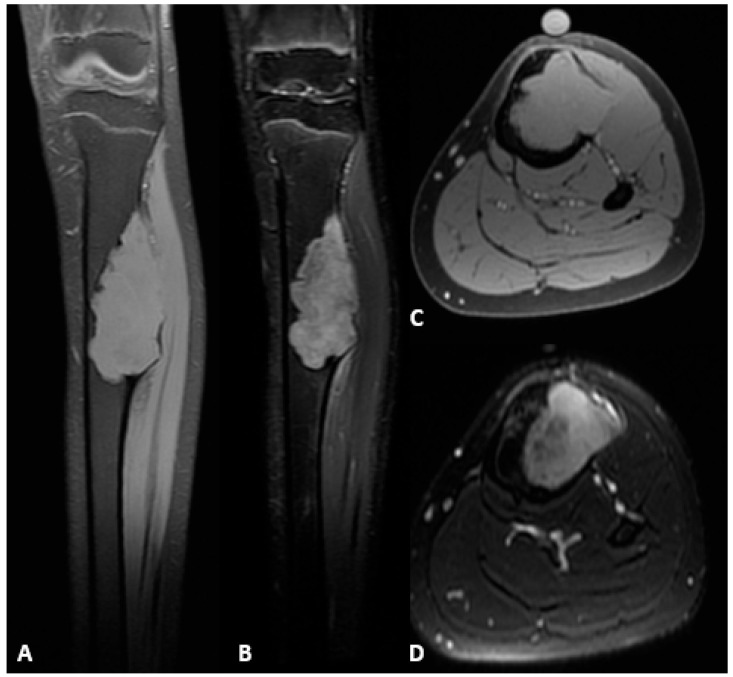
Preoperative MRI of the left tibial diaphysis demonstrating an OFD/LA. (A) Hypointense lesion on T1-weighted sequence, coronal plane. (B) Hyperintense lesion on T2-weighted fat-suppressed sequence, coronal plane. (C) Hypointense lesion on T1-weighted sequence, axial plane. (D) Hypointense lesion on T2-weighted sequence, axial plane.
